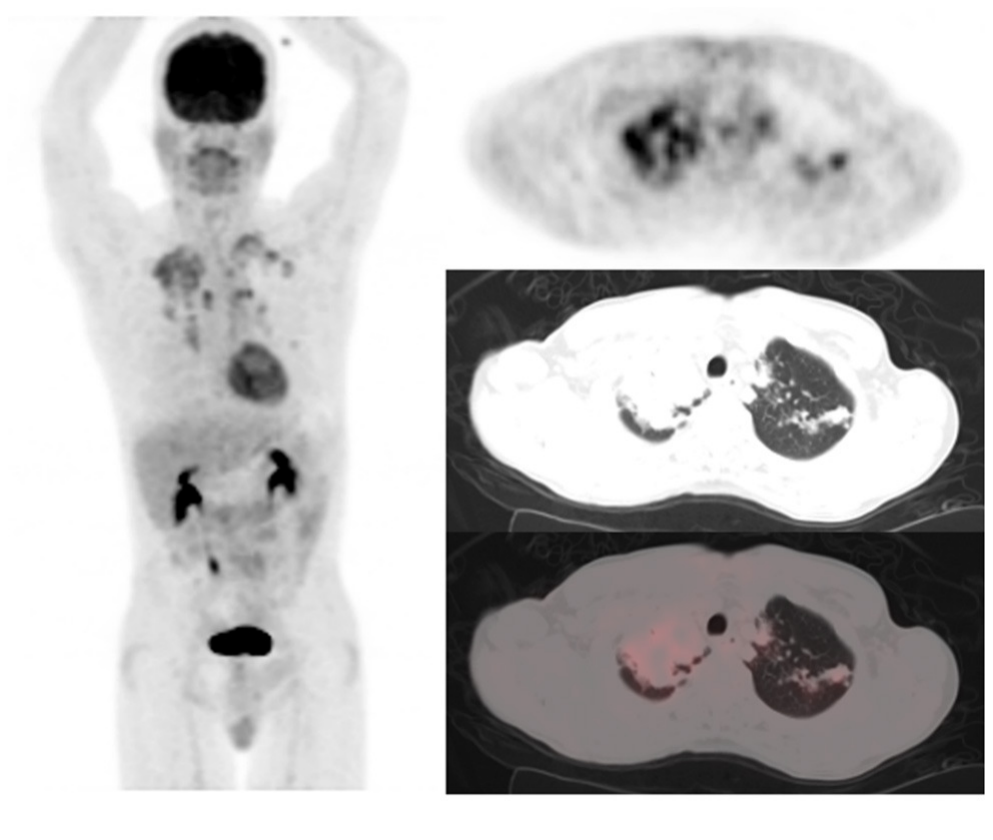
A 49-year-old HIV-infected male who completed a 6-month course of anti-tuberculous treatment. FDG PET/CT obtained for treatment response assessment. The maximum intensity projection scan (MIPS) on the left shows residual metabolic activity in the lungs bilaterally, mostly in the upper lobes, right more than left. The left column of images are the PET, CT, and fused PET/CT transverse section through the upper lobes of the lungs showing consolidation of right upper lobe parenchyma and left upper lobe lung nodules all showing residual metabolic activity post-treatment for pulmonary tuberculosis.
